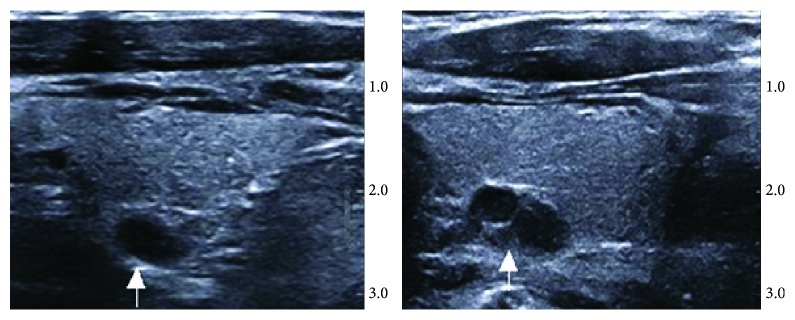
Conventional ultrasound images of parathyroid hyperplasia. In drug susceptible group, ultrasound images showed PTG with slight heterogeneousness. In drug insusceptible group, ultrasound images showed PTG with high heterogeneousness.
